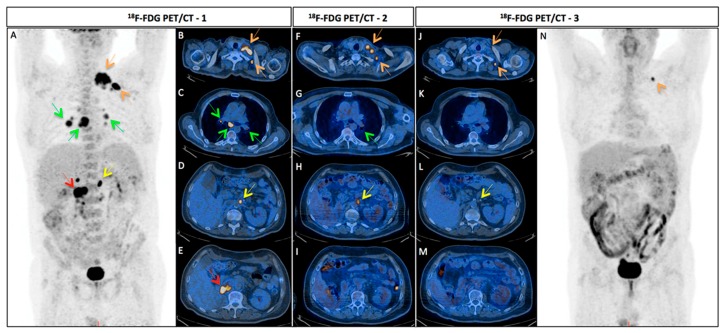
^18^F-FDG PET/CT TKIs therapy evaluation in a 52-year-old man affected by metastatic RCC six months after surgery resection of the right kidney. The ^18^F-FDG PET/CT-1 [MIP (A); and axial fused PET/CT (B–E)] detected several cervical (SUVmax 17.7, orange arrows), mediastinal (SUVmax 13.2, green arrows) and abdominal (SUVmax 16.7, yellow arrow) lymph nodes (A–C), as well as recurrent disease in right renal loggia (SUVmax 11.9, red arrow) (E) at baseline. The ^18^F-FDG PET/CT-2 [axial fused PET/CT (F–I)] performed after 1 cycle of TKIs demonstrated partial but early disease response with persistent ^18^F-FDG uptake in cervical (SUVmax 12.2-orange arrows), mediastinal (SUVmax 3.4, green arrows) and abdominal (SUVmax 6.7, yellow arrow) lymph nodes (F–H) and increased metabolism in right renal loggia (I), despite only slight reduction in size. In the ^18^F-FDG PET/CT-3 [MIP (N); and axial fused PET/CT (J–M)] performed after six months of TKIs therapy, only a persistent cervical (SUVmax 6.8, orange arrows) lymph node (J) was detectable. Other lymph nodes were still recognized on CT examination but they were glucose metabolism-free ((J) upper orange arrow; and (L) yellow arrow). ^18^F-FDG PET/CT, 18F-fluoro-2-deoxy-2-d-glucose positron emission tomography/computed tomography; TKIs thyrosin kinase inhibitors, RCC, renal cell carcinoma.
